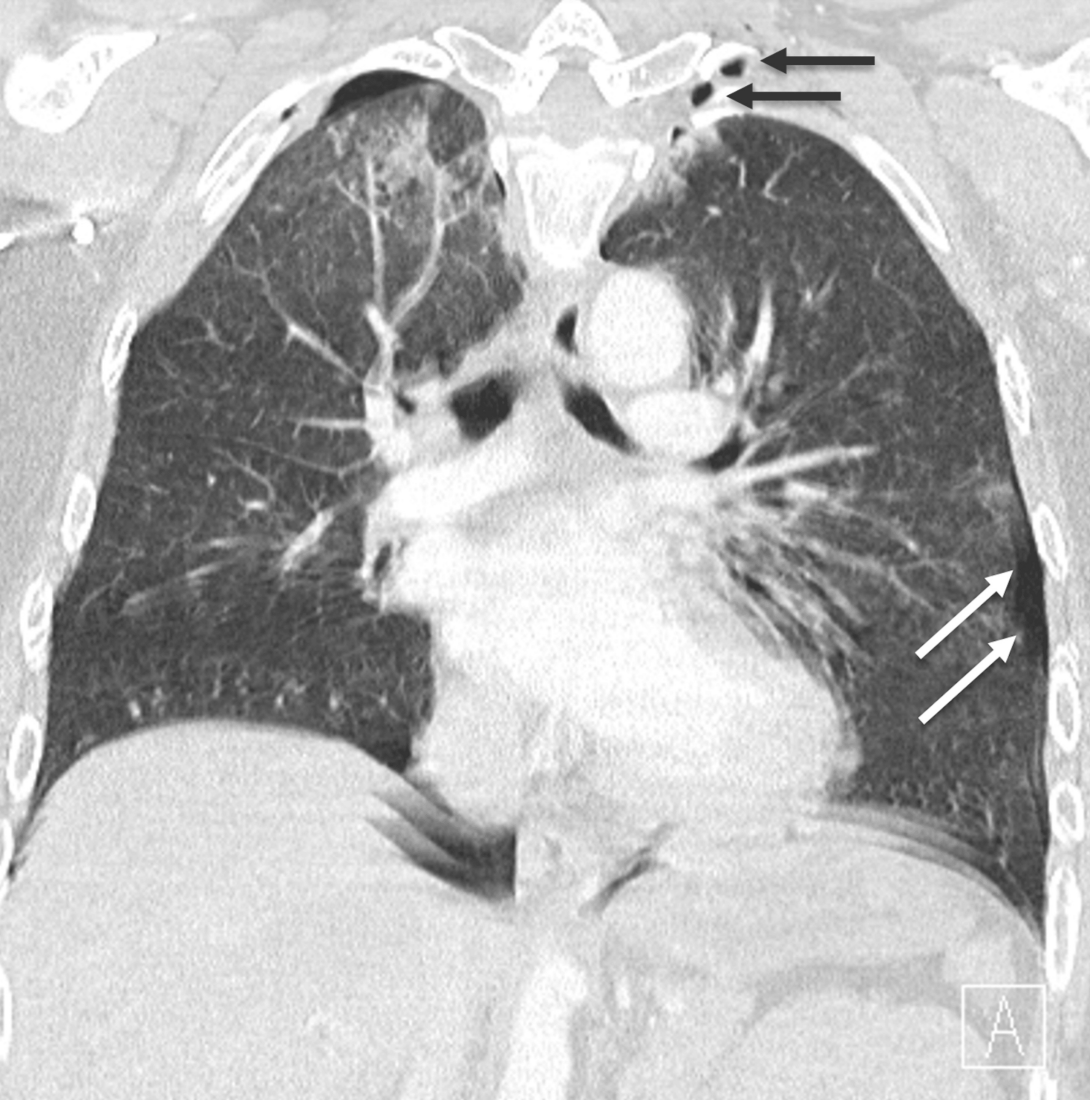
Chest computed tomography showing small left pneumothorax (white arrows) and gas in soft tissues adjacent to lateral masses (gray arrows).
